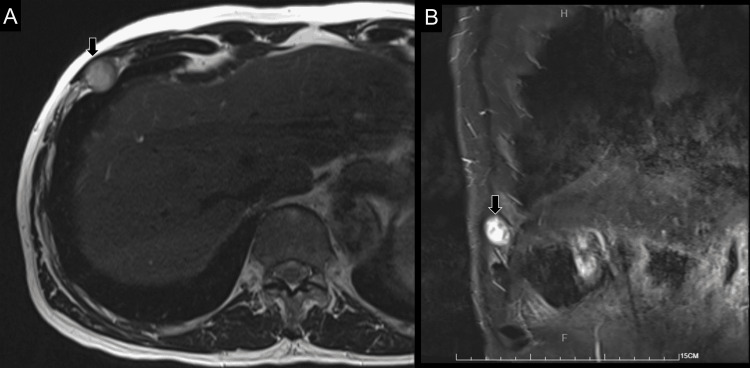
T2-weighted MRI of the intercostal tumor (arrows) in the axial (A) and coronal (B) views.
